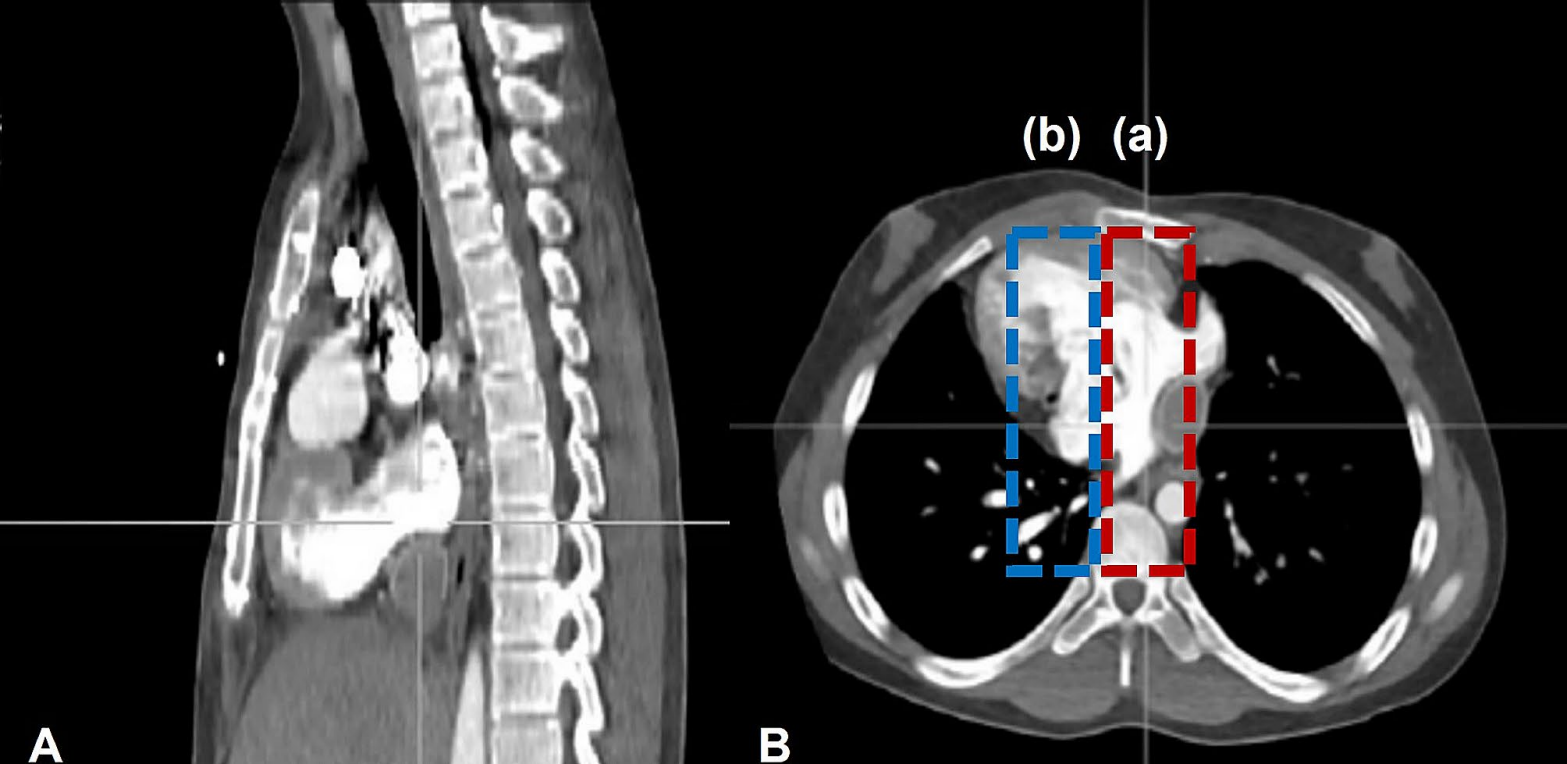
DORV with dextrocardia in a male aged 13 years. Measurement of areas on chest CT images. After confirming the position of the lower quarter of the sternum in the sagittal section (A), the area of ​​each heart structure was measured in the corresponding axial section (B). In Fig. 3B, (a) the predicted compressed area of each structure of the heart behind the sternum (the red dashed line) at the same level and (b) the predicted compressed area of each structure of the heart after moving the width of the sternum at the right costosternal junction (the blue dashed line) are shownDORV, Double outlet right ventricle
